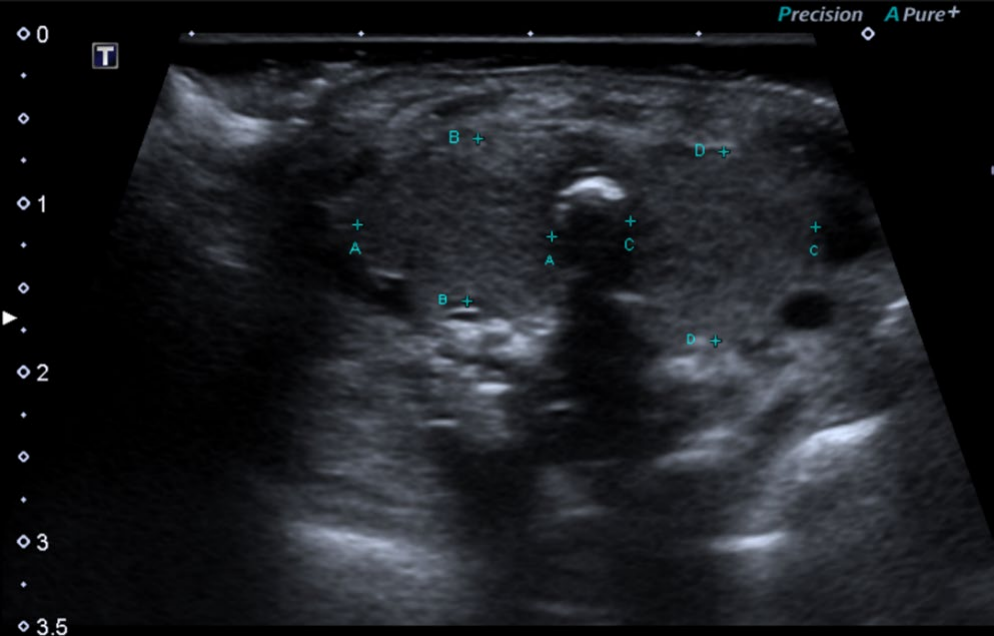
Postnatal thyroid gland ultrasound demonstrates diffuse enlargement.
